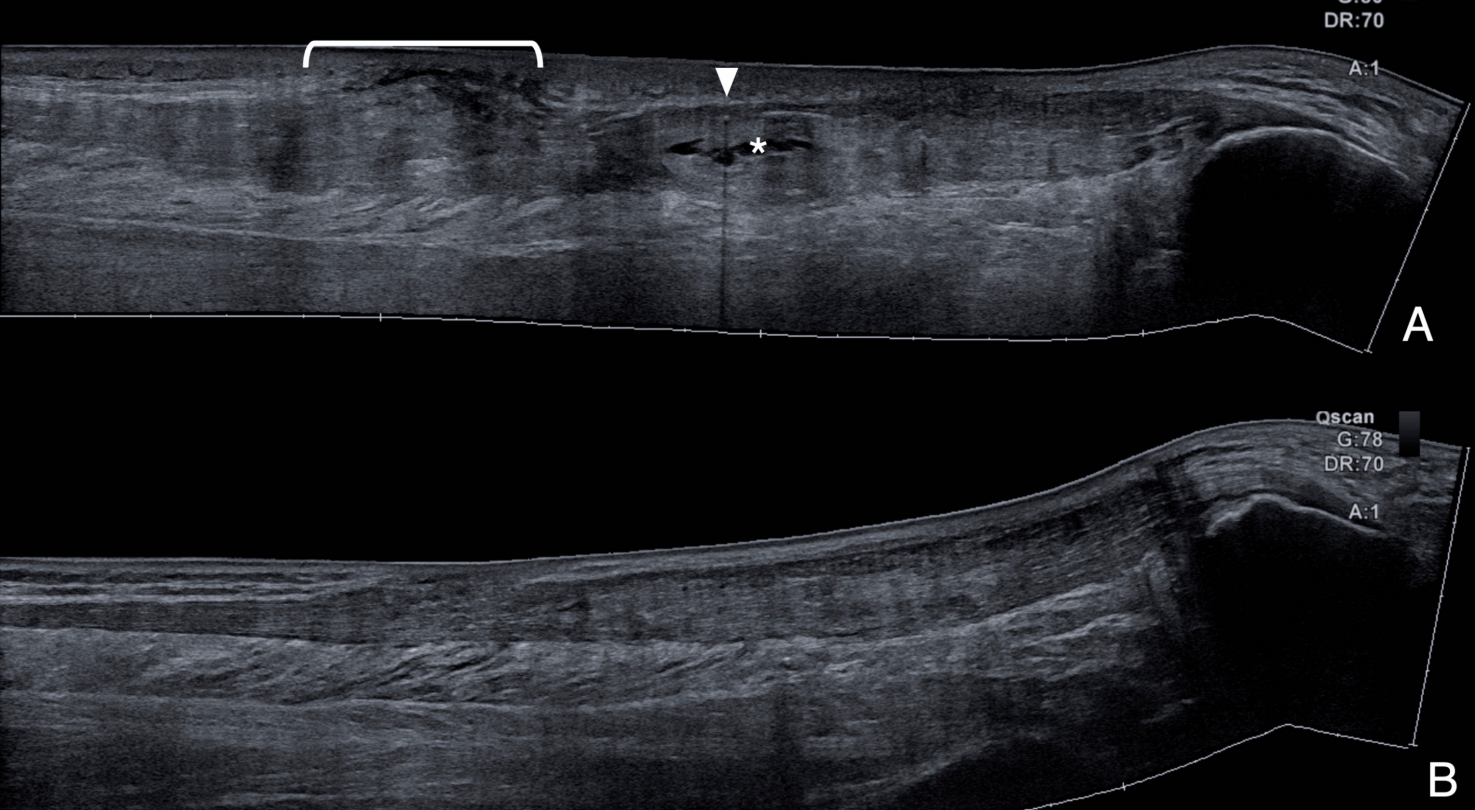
Ultrasound showing restoration of normal post-tenorrhaphy status (Patient 1) A) Pre-suture removal B-mode ultrasound extended view reveals a cystic-like lesion (white asterisk) adjacent to the suture path (white arrowhead) and subcutaneous inflammatory changes (white bracket). B) Post-suture removal B-mode ultrasound extended view shows restoration of normal post-tenorrhaphy status.
